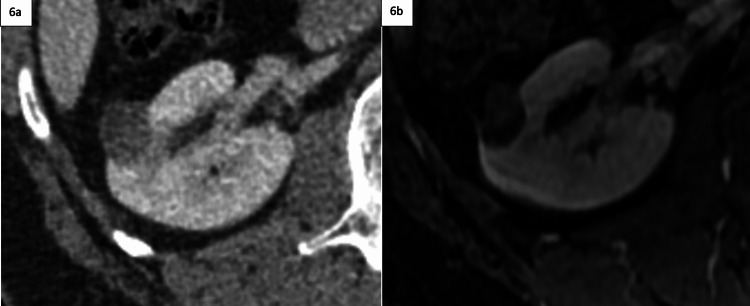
Enhanced CT showing indeterminate lesion with follow-up MRI 8 months later 6a: Contrast-enhanced CT ordered in the emergency room for right lower quadrant pain, demonstrating an incidentally noted indeterminate right renal lesion measuring less than 1.9 cm. The radiologist recommended a follow-up MRI in 6-12 months, utilizing the renal lesion follow-up macros. 6b: Contrast-enhanced abdominal MRI, ordered on an outpatient basis 8 months later demonstrates an enhancing renal malignancy without significant interval growth or invasive features.
